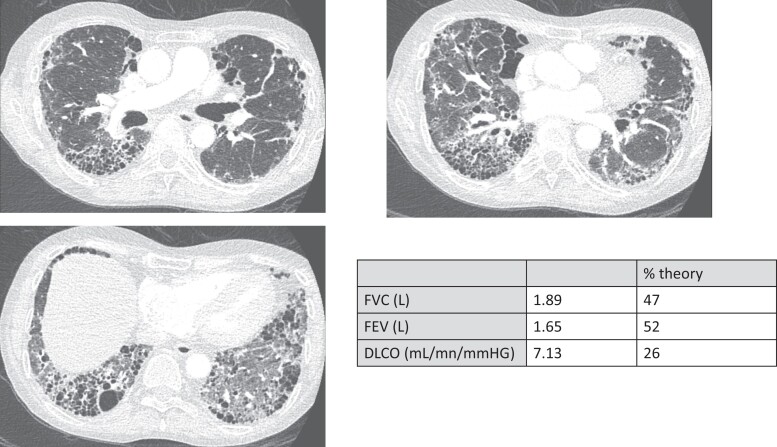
Patient #25 chest computed tomography scan and lung function tests. Bronchiectasis, honeycombing, micro and macrocystic parenchymal destruction, and ground-glass opacities. Abbreviations: FVC, forced vital capacity, FEV, forced expiratory volume in 1 second.
